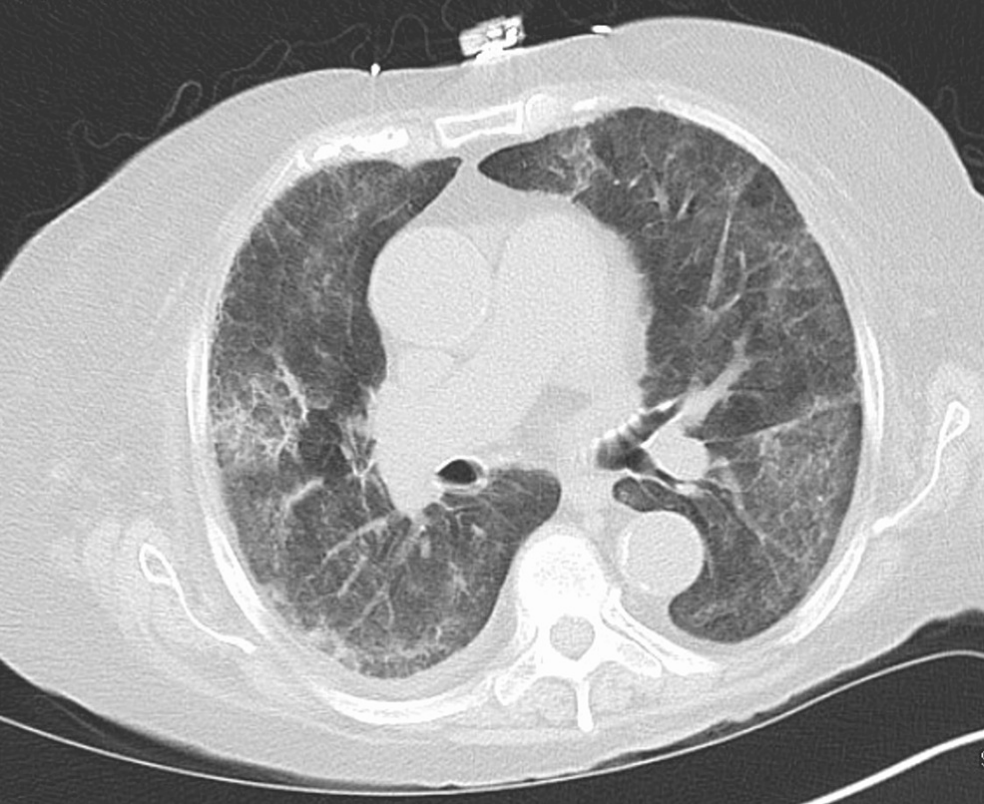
Follow-up CT chest without contrast showing worsening bilateral ground-glass densities in a mosaic distribution and inter and intralobular septal thickening CT: computed tomography
